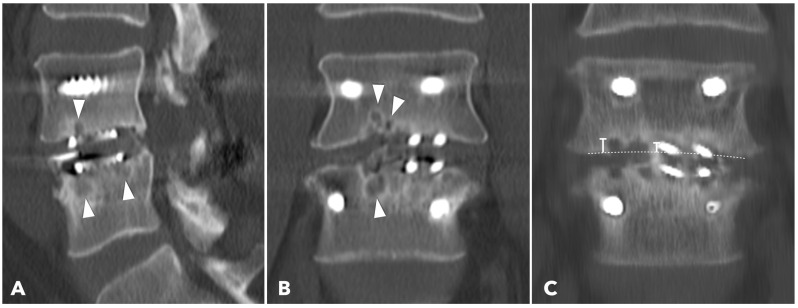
(A,B) 1-year CT reconstruction revealed the presence of subchondral osteolysis or endplate cyst formation (white arrowheads). (C) The measurement of cage subsidence on the coronal CT reconstruction image.
